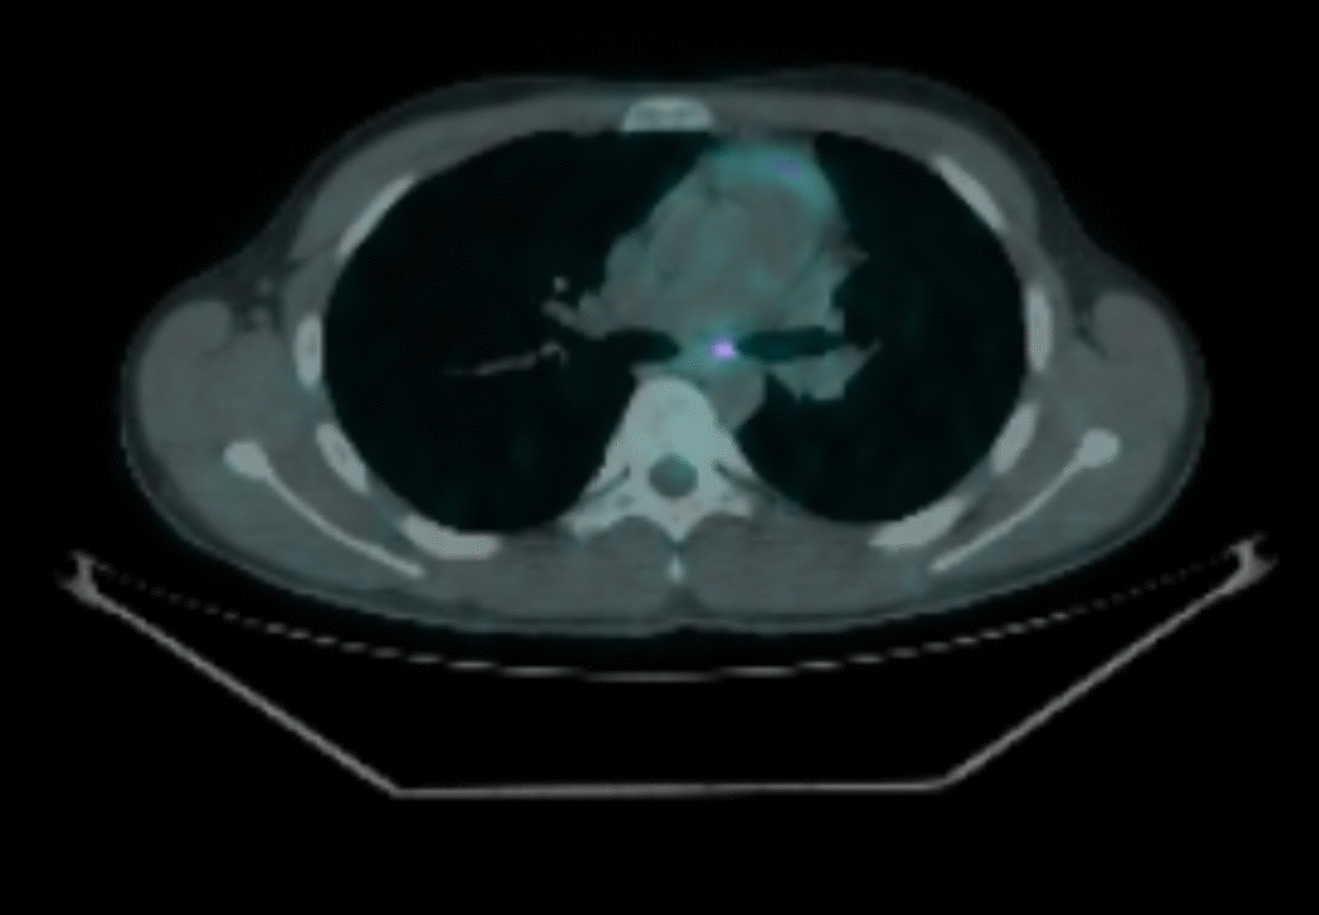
PET-CT showing subcarinal uptake at presentation
